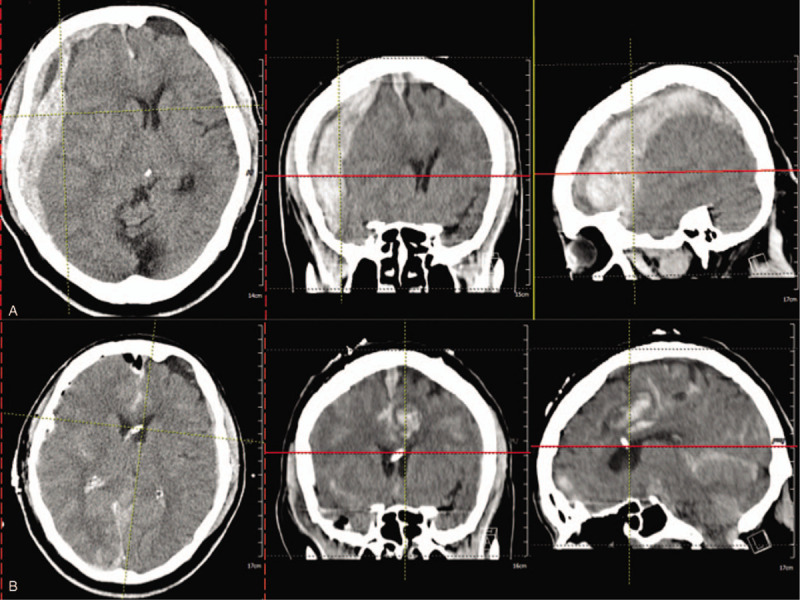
(A) Brain CT showed intra-cerebral hemorrhage with subdural hemorrhage and subarachnoid hemorrhage on 2014-12-21. (B) After craniotomy with hematoma evacuation, brain CT showed some residual hematoma still in the cerebral and cerebellar area on 2014-12-22. CT = computed tomograpghy.
